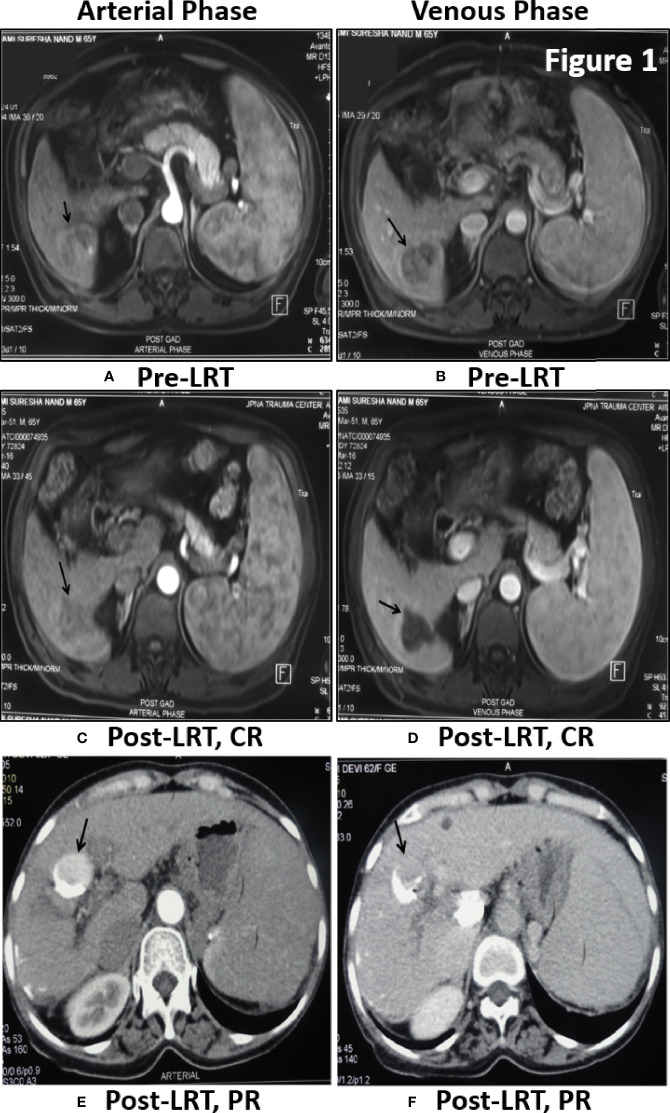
Tumor response assessment by MPCT/MRI of Liver: Post-TACE MRI Scan of 65 yr old male patient of HBV- HCC have shown large solitary HCC in segment 6 showing enhancement in arterial phase (A) with washout in venous phase (B) before treatment. One month Post-LRT in MRI have shown lack of enhancement in treated tumor in arterial phase (C) and venous phase (D) is suggestive of Complete Response (CR). Post-TACE CT scan of 62 yr old female patient of HCV-HCC have shown residual enhancing tumor (black arrow) in arterial phase (E) with washout in venous phase (F). Dense lipidol seen in the lateral aspect of tumor is suggestive of partial response to LRT.
